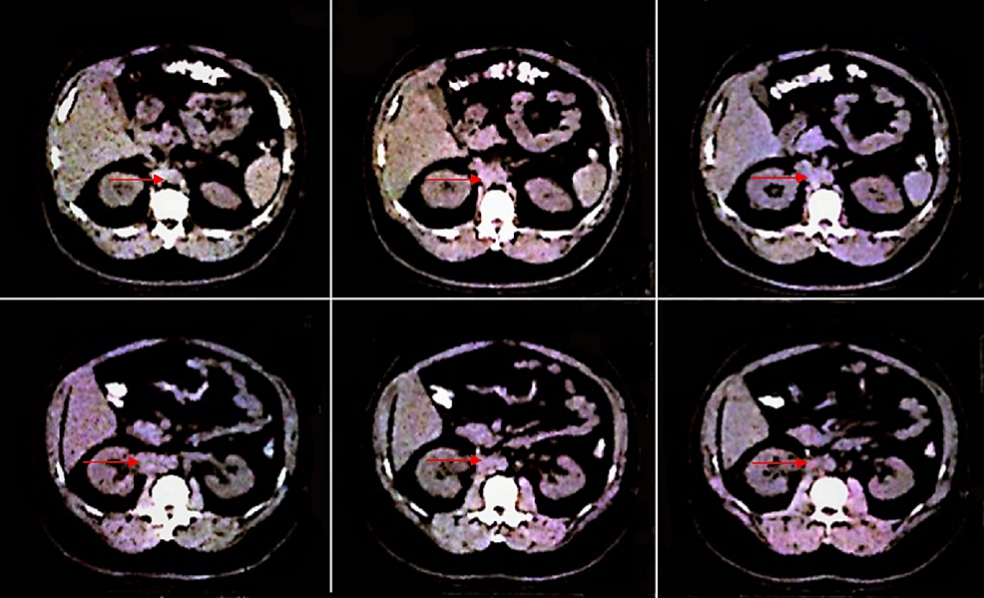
CT-scan of the abdomen and pelvis after four cycles of chemotherapy with BEP showed a significantly smaller yet persistently enhancing retroperitoneal mass. CT: computed tomography. BEP: bleomycin, etoposide, and cisplatin. Red arrows point to the remaining retroperitoneal mass post-chemotherapy.
